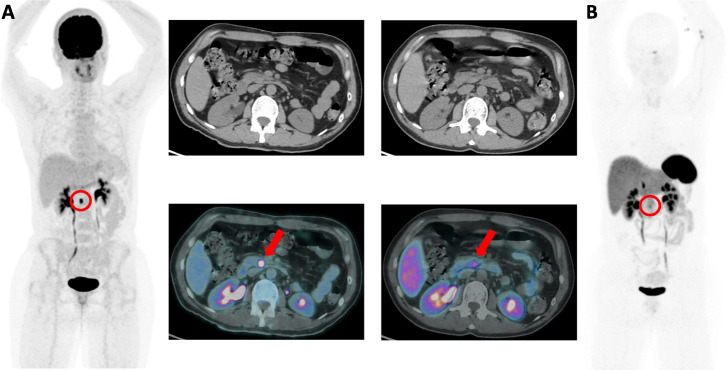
a Initial 18F-FDG PET demonstrated a high metabolic expression in the uncinate pancreatic nodule (SUV_max_ 16.4), apparently stable in size when compared to previous exams. b 68Ga-DOTATOC shows the same nodule in the uncinate process of the pancreas with low somatostatin receptor expression (SUV_max_ 9.2), poorly characterized in the image without contrast
